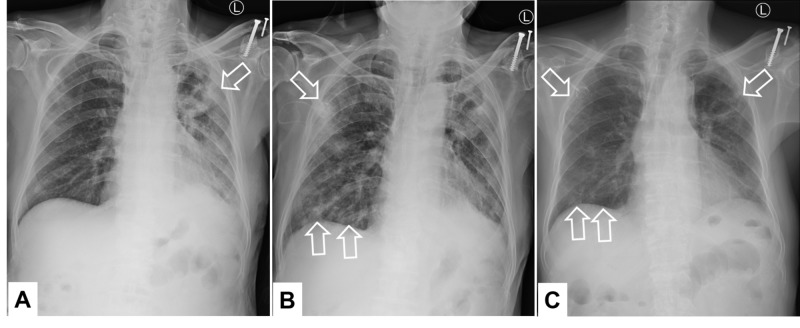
Chest X-ray films along the clinical course: A. May 29, 2019. B. June 10, 2019. C. June 20, 2019 Arrows: Targets of interest
